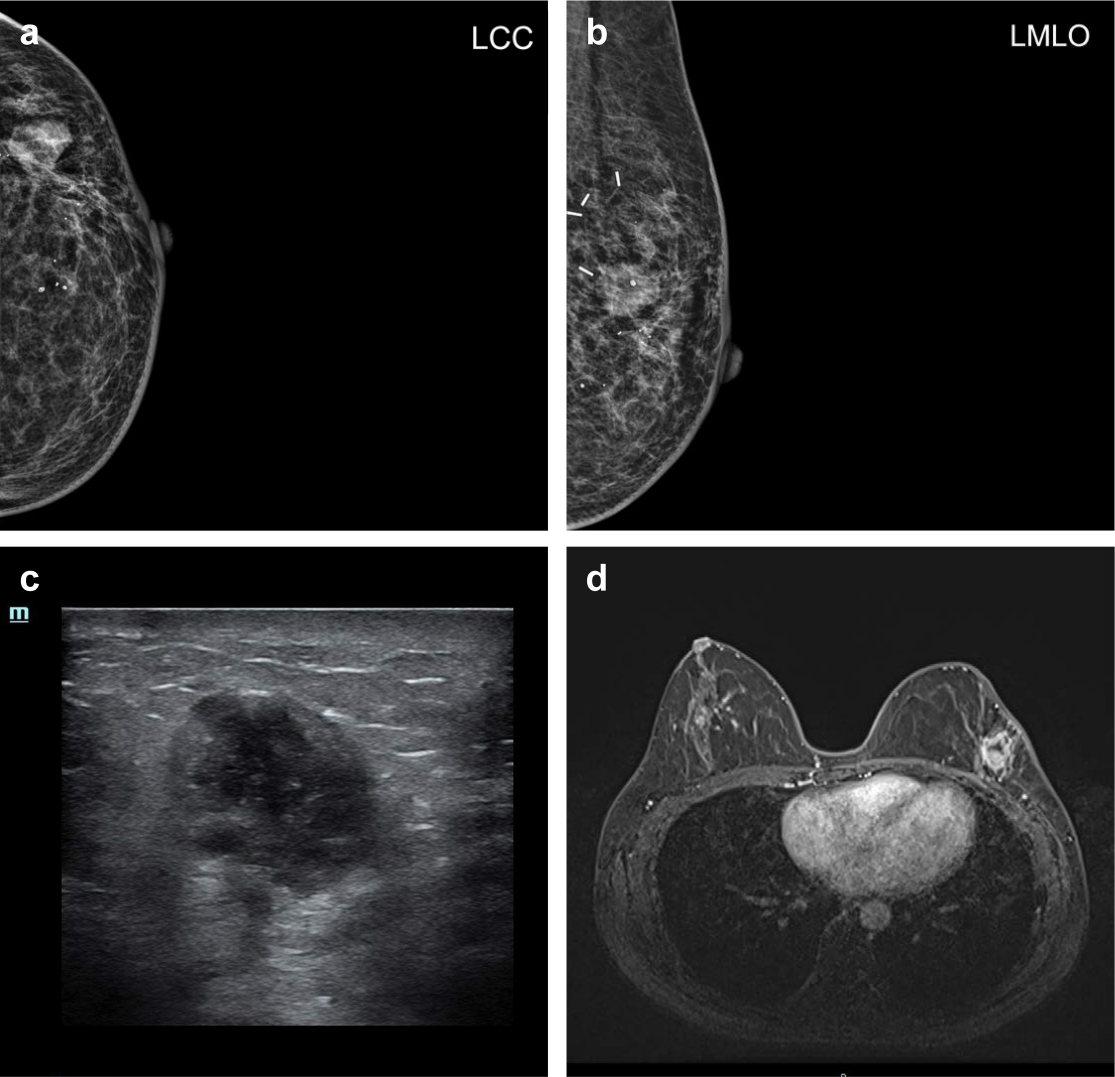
Radiology. (A) Left craniocaudal (LCC) view and (B) left mediolateral oblique (LMLO) view of mammography. (C) Representative ultrasound imaging of a breast lesion. (D) Representative MRI imaging of a breast lesion.
